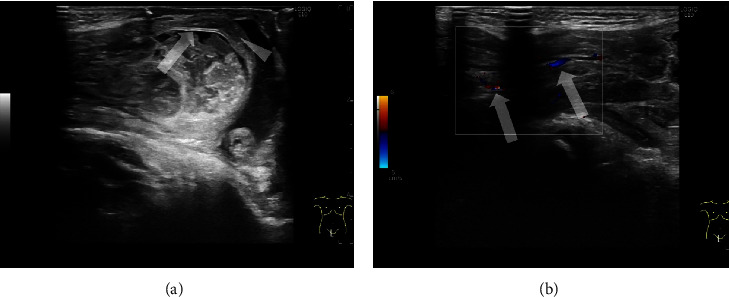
Case 1: an 11-month-old male with a right incarcerated inguinal hernia. (a) Sonogram of the right inguinal bulge showing a cystic structure accompanying intestinal wall layers. A high-echoic layer as mucosa (arrow) and a low-echoic layer (arrowhead) as submucosa or muscular layer can be visualized. Due to edematous changes in the intestinal wall, the five-layer structure of the intestinal wall could not be clearly visualized. (b) Color Doppler sonogram of the inguinal hernia shows vascular flow within the intestine (arrows). Intestinal peristalsis was preserved but weak.
